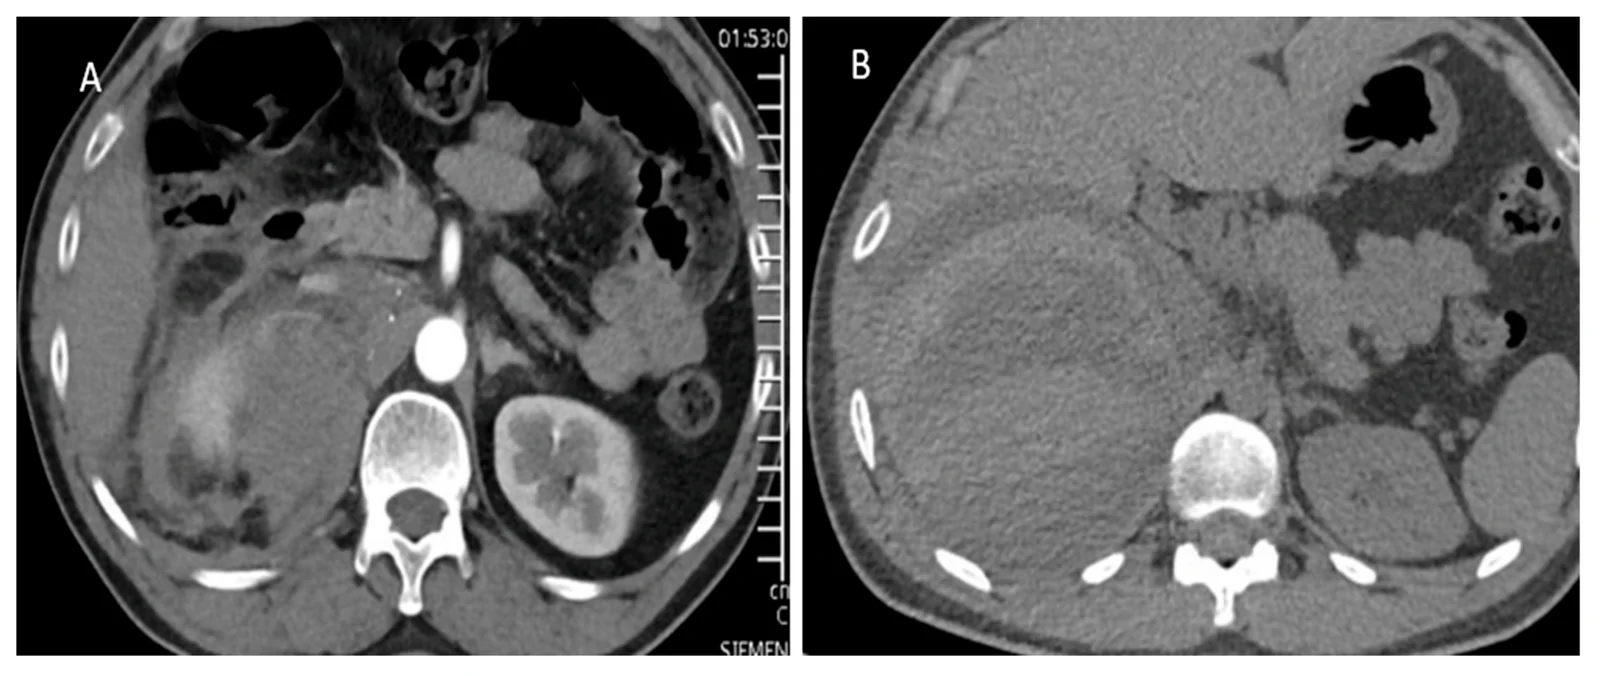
(A): CT abdomen with contrast: Enlarged right adrenal gland with an ill-defined peripherally; enhanced right adrenal mass and perilesional/retroperitoneal hematoma extending to the perinephric space (Case 5). (B): CT urinary tract: A huge heterogeneous collection is seen in the right upper quadrant extending down to the lower abdomen. Measuring 15 × 12 cm, it is heterogenous, indicating a hemorrhagic component (Case 7).
